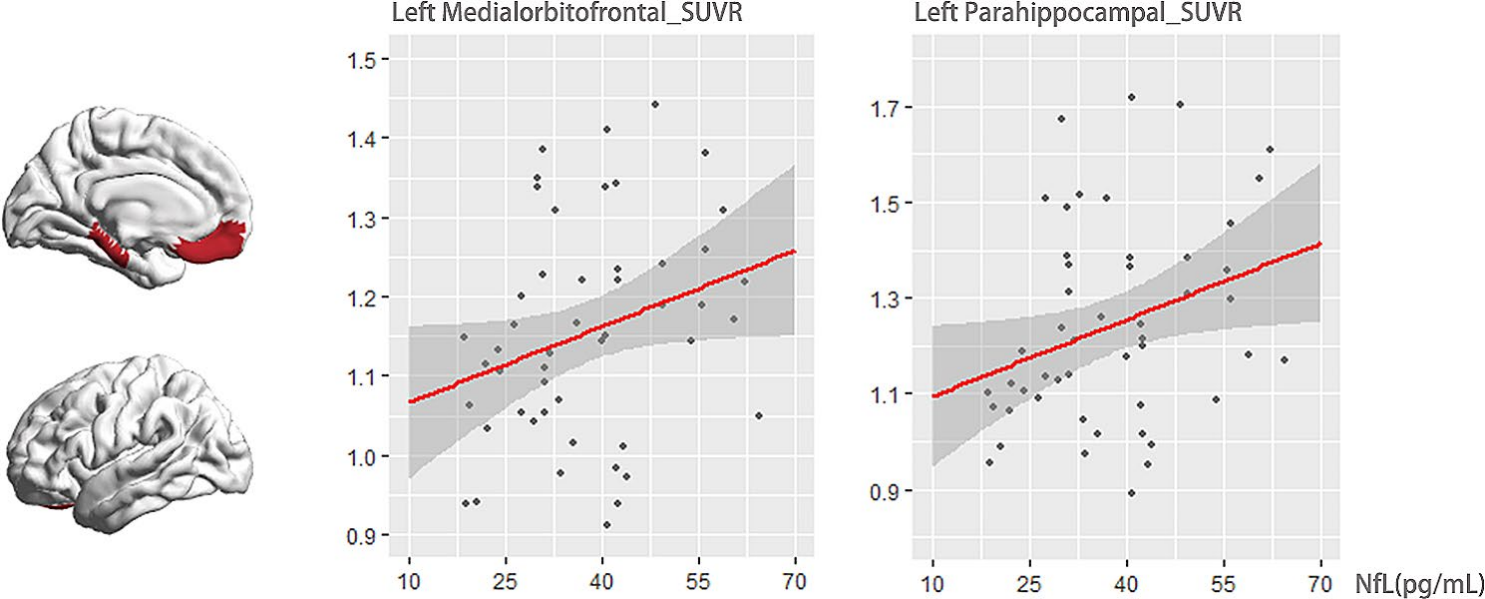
Association of plasma NfL with flortaucipir uptake after approximately 5 to 7 years. Among the 50 Aβ + CN and MCI participants with tau PET data available, multivariable linear regression analysis was performed to explore the predictive value of baseline plasma NfL concentrations for future tau deposition in the brain. Regional tau burden after 5 to 7 years of follow-up was used as the dependent variable and baseline NfL as a predictor, controlling for age, sex, and years of education. The baseline NfL concentration and SUVR PET uptake in the left medial orbitofrontal cortex and para-hippocampus were positively correlated (F = 2.474, *P*<0.029; F = 2.224, *P*<0.042). Abbreviations: NfL, neurofilament light protein; SUVR, standardized uptake value
